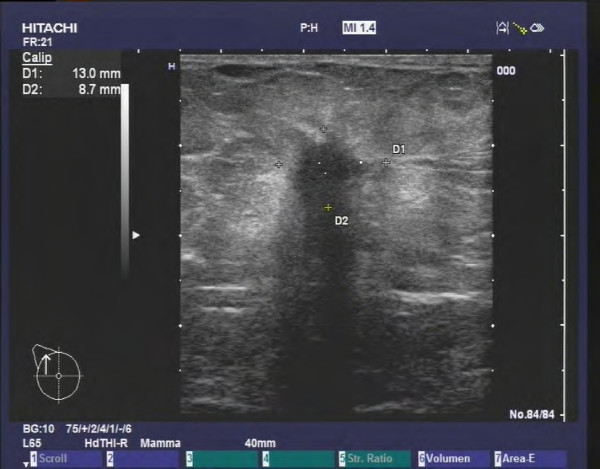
Invasive ductal carcinoma in a 72 year old patient (HR positive, Her2 negative, G2). The tumor exhibits typical ultrasound criteria for malignancy (irregular, hypoechoic mass with an indistinct, spiculated margin, an echogenic halo, posterior shadowing and architectural distortion of the surrounding tissue).
